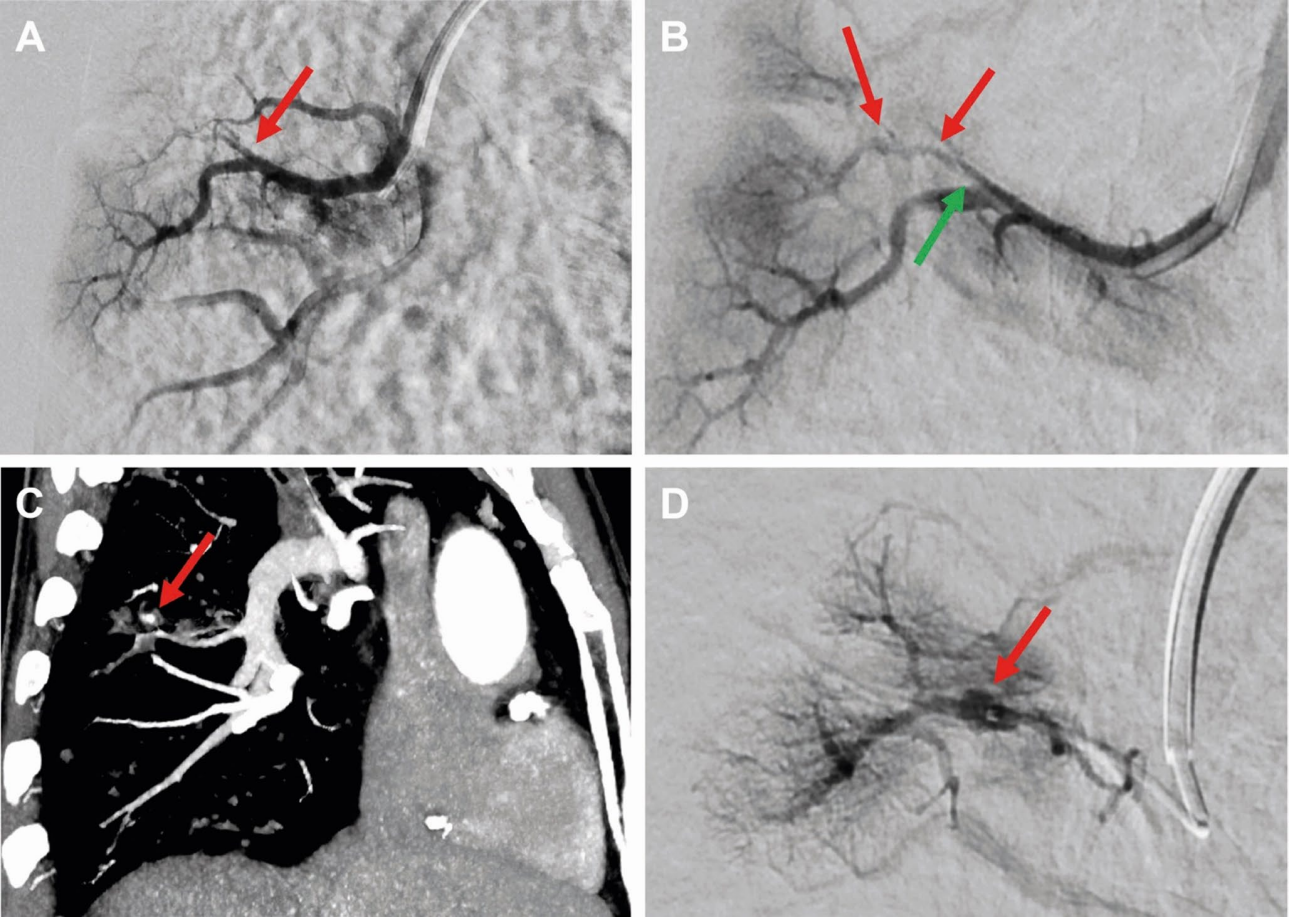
Imaging of pulmonary artery embolization in a patient with Rasmussen aneurysm. A Digital subtraction angiography (DSA) showing the right lower pulmonary artery and the extensive alterations of the subsegmental branch without depiction of a Rasmussen aneurysm (red arrow). B DSA after microcatheter placement proximal to the aneurysm bearing subsegmental branch (green arrow). Again, the aneurysm is not depicted (red arrow). C Contrast-enhanced CT angiography (CTA) in maximum intensity projection of the right lower pulmonary artery, here, the red arrow highlights the cavitary lesions with adjacent Rasmussen aneurysm. D DSA images after microcatheter placement in the subsegmental branch verify the Rasmussen Aneurysm in the suspected subsegmental branch
